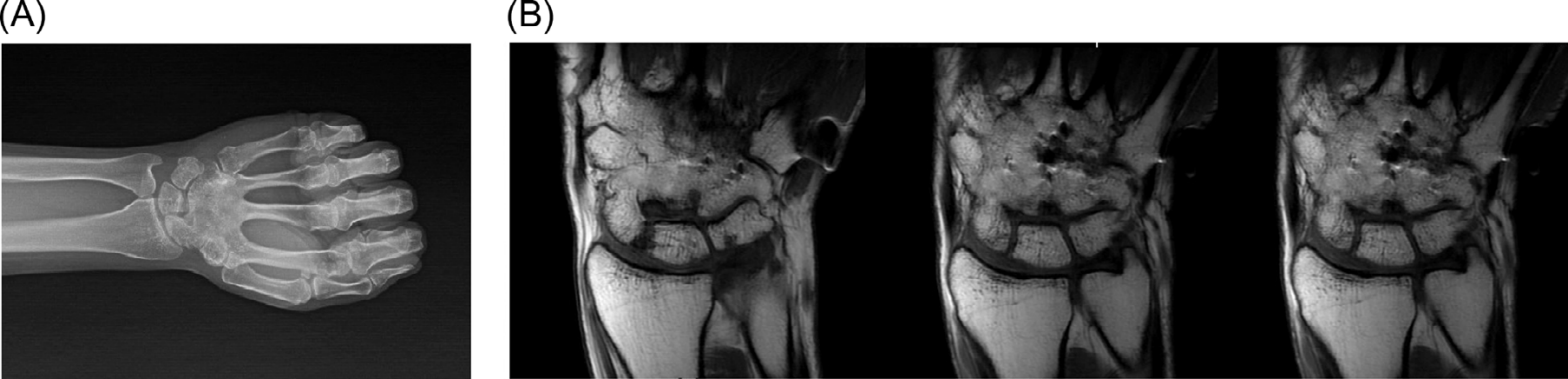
(A) Roentgenogram of the operated wrist at 10-year follow-up, (B) MRI of the operated wrist at 10-year follow-up.
